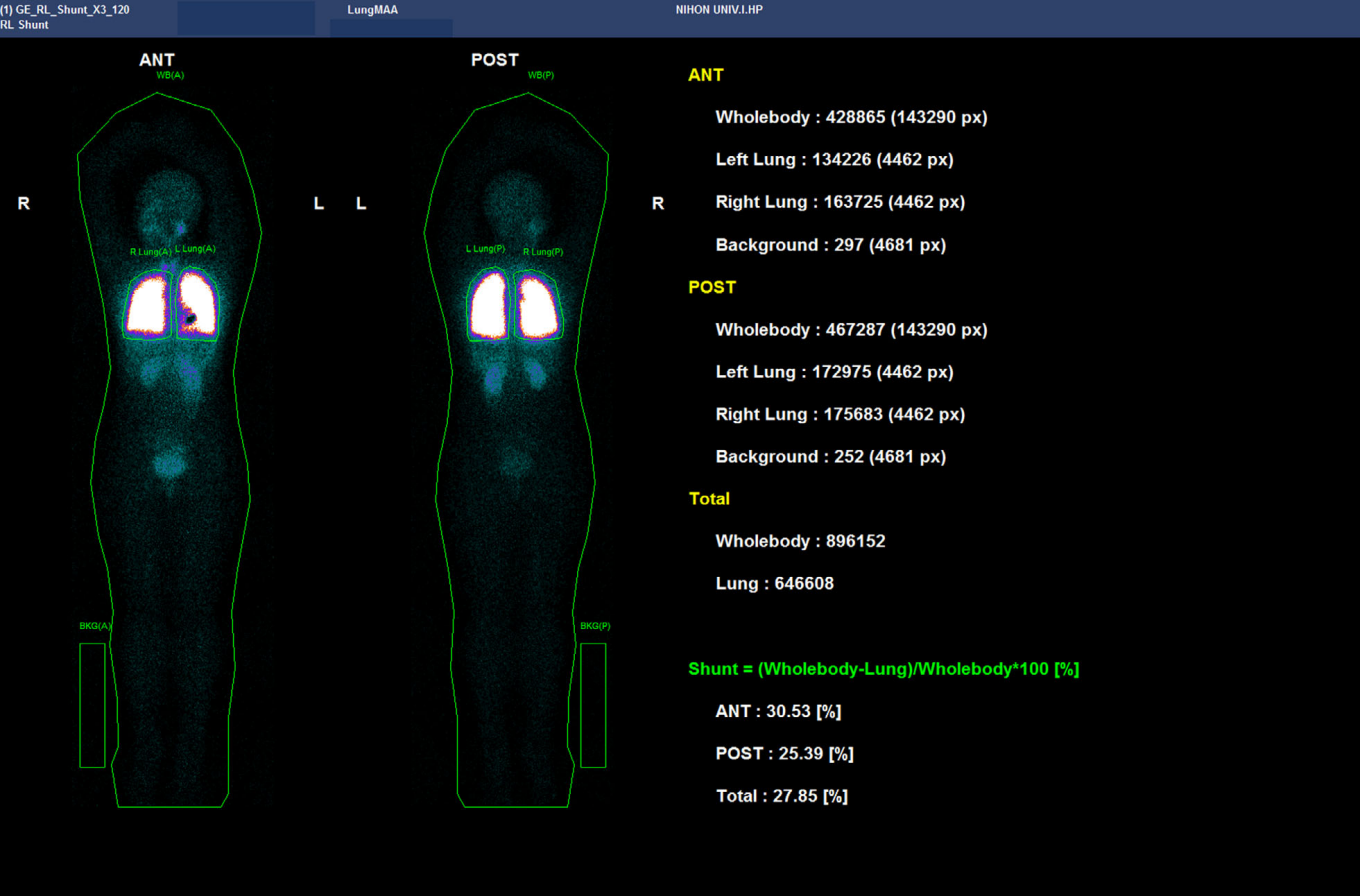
Lung perfusion scintigraphy. Uptake in the kidneys suggested an intrapulmonary right‐to‐left shunt. Shunt rate: 27.9%
